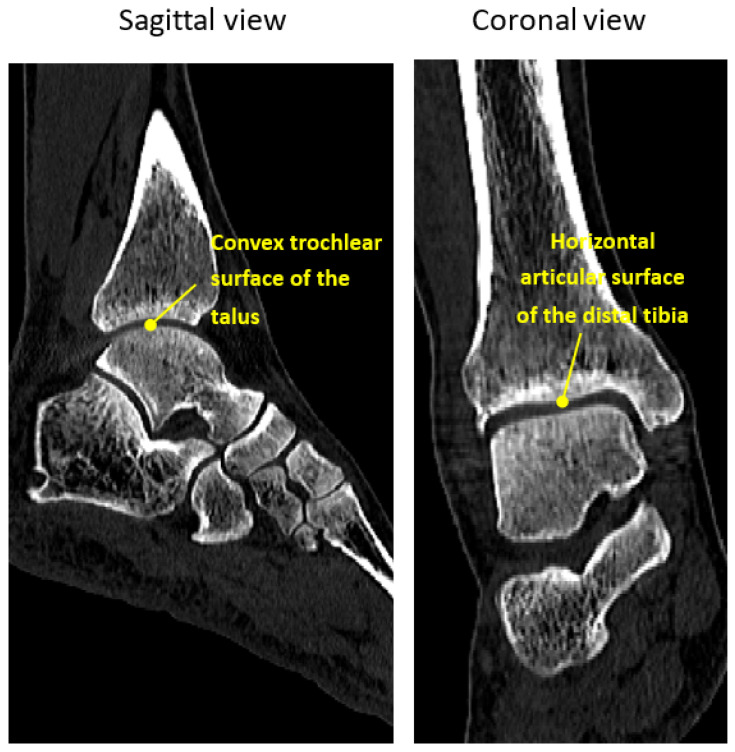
Sagittal and coronal views of the ankle CT image.
